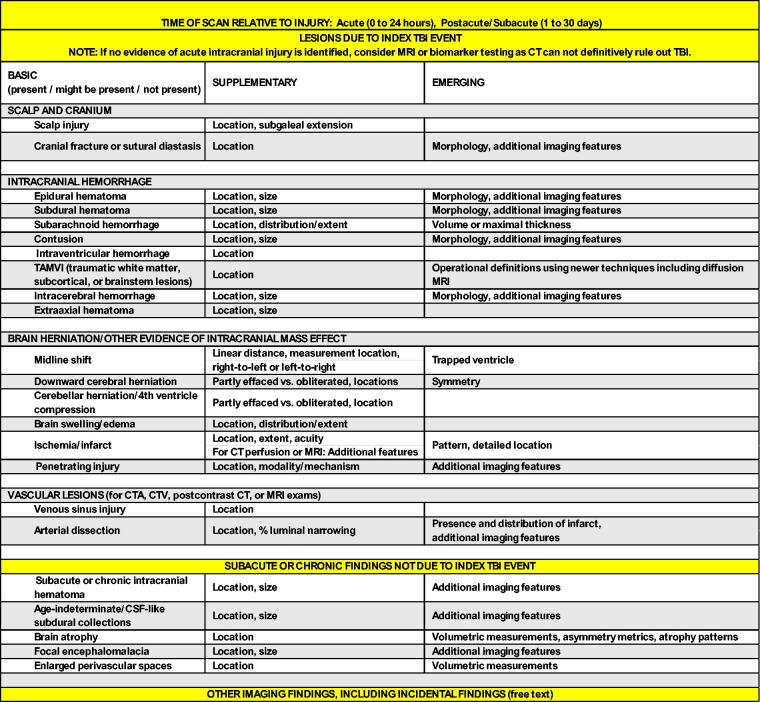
CT, computed tomography; TAMVI, traumatic axonal and/or microvascular injury; TBI, traumatic brain injury; CSF, cerebral spinal fluid.
